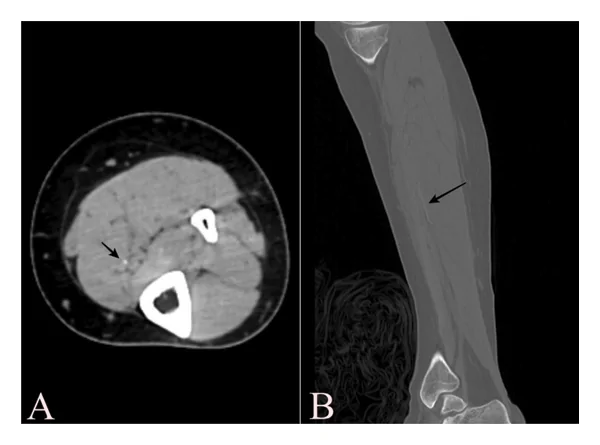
(A) Axial CT image demonstrating the catheter fragment at the mid‐cruris level. (B) Sagittal CT image showing the displaced catheter fragment extending proximally from the original insertion site.
